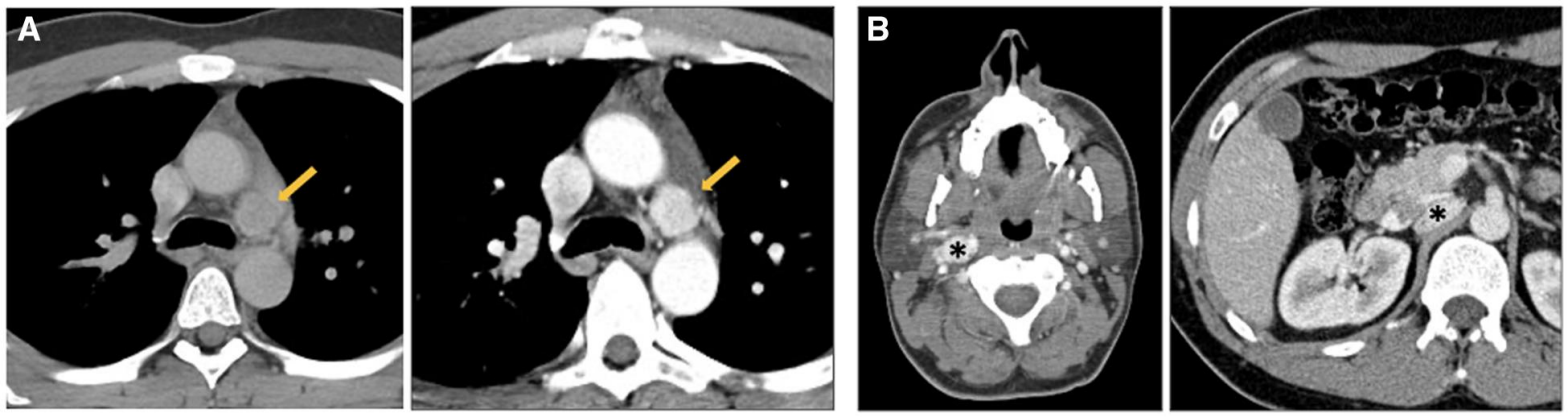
Paraganglioma. (A) Plain (left) and contrast-enhanced axial CT (right) shows a well-defined hypodense lesion (arrow) in the visceral mediastinum (aortopulmonary window) with intense contrast enhancement. (B) Multiple paragangliomas have been identified in other sites of the same patient: at the division of the right common carotid artery (left image) and retrocaval (right image).
